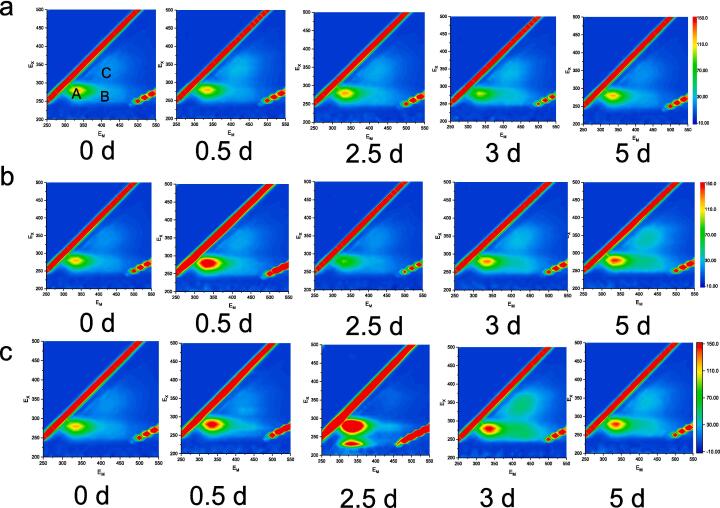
Excitation-Emission Matrix (EEM) of Extracellular Organic Matter (EOM) Changes. (a) Control; (b) Single ultrasound treatment; (c) Second ultrasound treatment. The single ultrasound treatment consisted of a 20-minute session on the first day. The second ultrasound treatment involved a subsequent 20-minute session on the 2.5th day, following the initial treatment.
